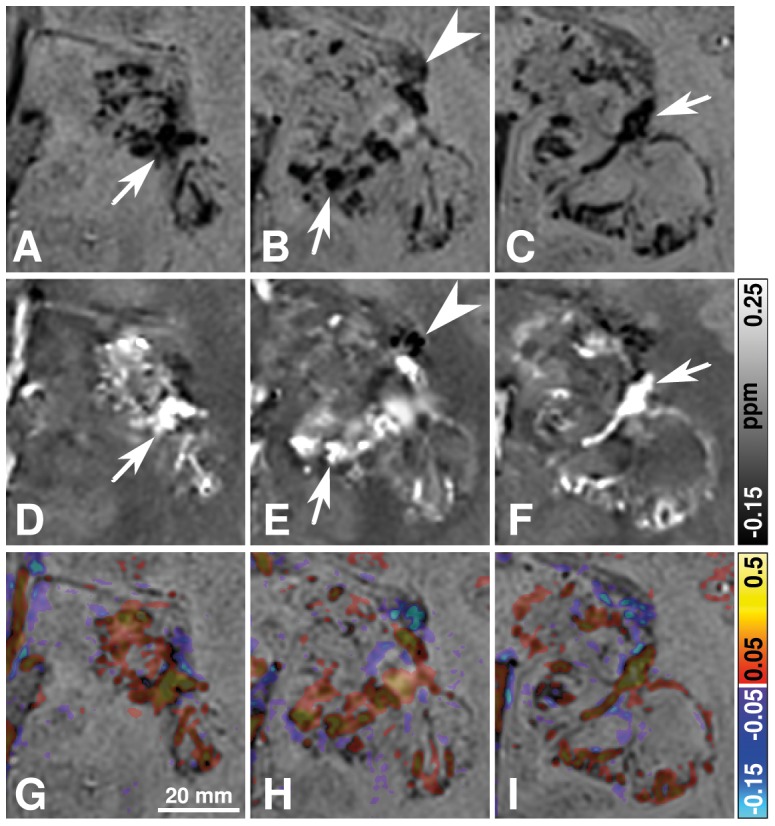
Sections of MR images of the glioblastoma presented in Figure 2. The top (a–c), middle (d–f), and bottom row (g–i) display sections of SW images, susceptibility maps, and overlays of the susceptibility maps onto the corresponding SW images, respectively. The columns from left to right sample the glioblastoma from inferior to superior direction with a slice distance of 2.5 mm. The location of these sections is indicated by the white rectangle in Figure 2c. The arrows and arrow heads indicate SBS and choroid plexus, respectively.
